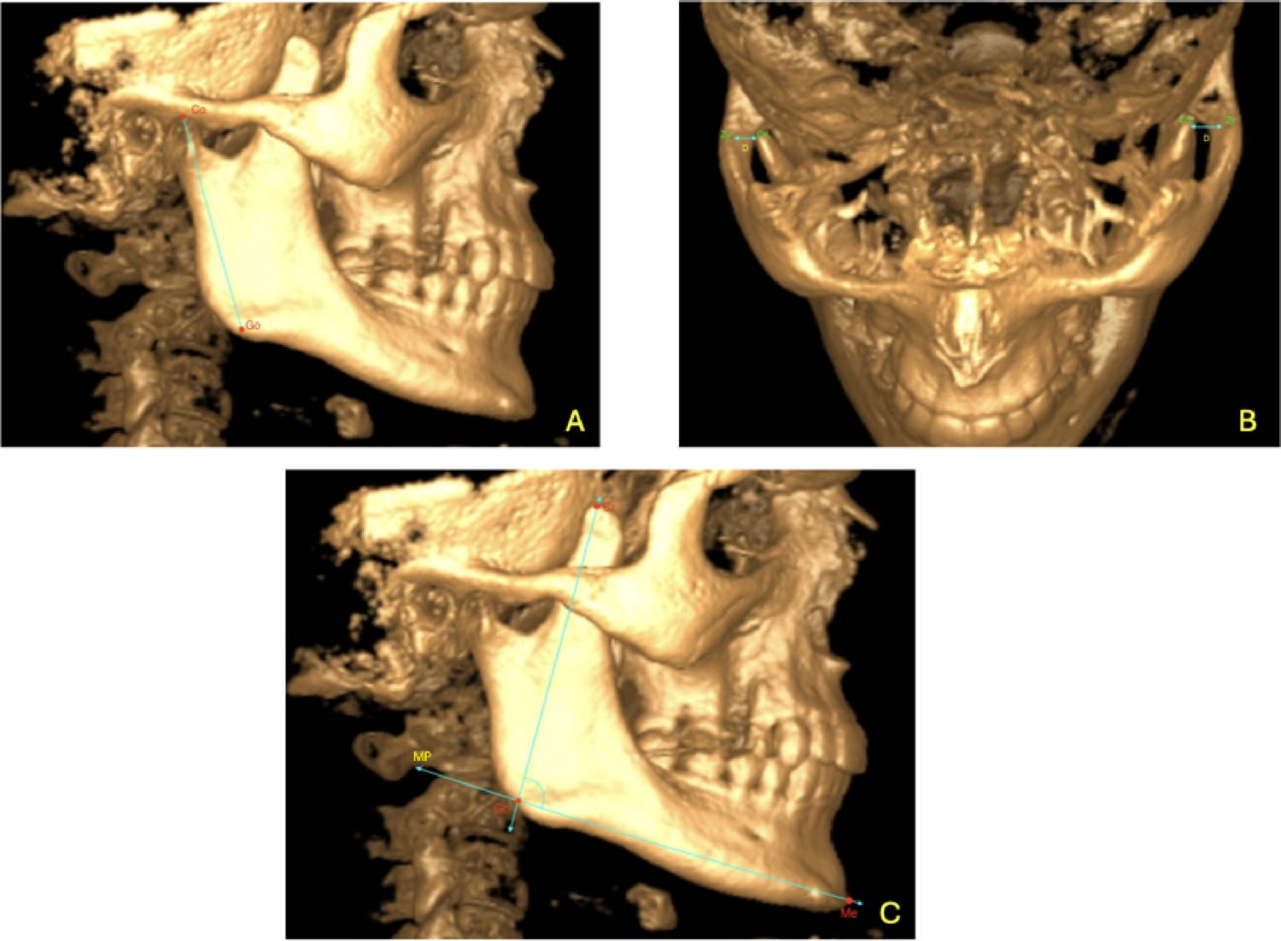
Sagittal and axial views of the CBCT scans (a Ramus length (H4), b Distance between the coronoid process, and the zygomatic arch (D), c Angle between the coronoid process and the mandibular plane (Cr-MP))
